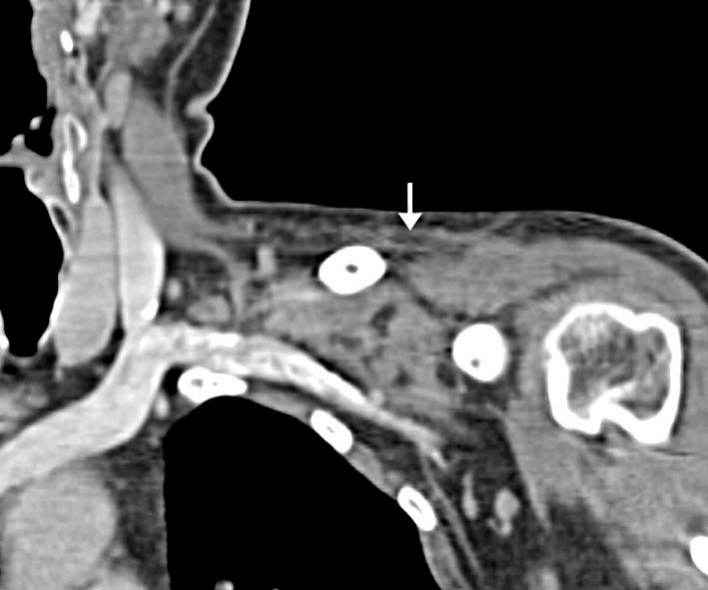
Coronal CT scan post-intravenous contrast following completion of antibiotic therapy (kVp 120, modulated mA). There has been almost complete interval resolution of the subcutaneous oedema, with minimal residual inflammatory fat-stranding in the supra- and infra-clavicular spaces.
